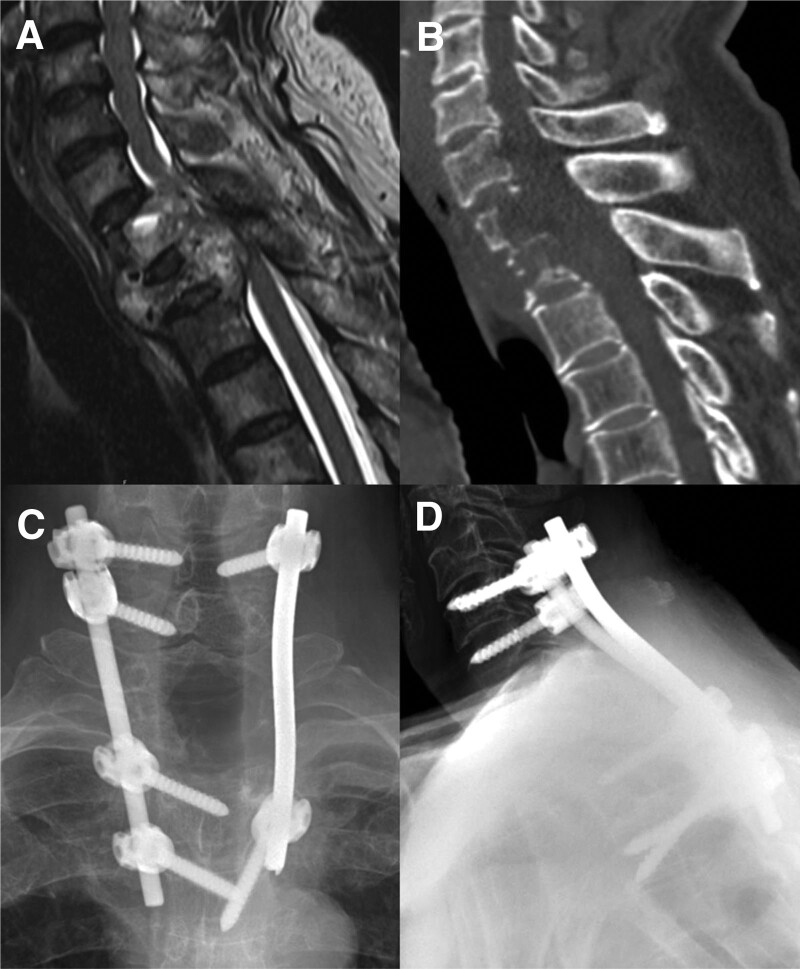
A 64-year-old man diagnosed with kidney cancer presented to the hospital with gait disturbance and severe back pain. Preoperative sagittal MRI (A) and sagittal CT (B) reveals the osteolytic metastatic spinal tumor at T1–2, compromising the spinal cord. Tumor removal with CTJF using 5.5-mm-diameter rods is performed from C6 to T4. Postoperative anteroposterior (C) and lateral (D) X-ray films show well-fixed pedicle screws at the cervicothoracic junction. CT = computed tomography, CTJF = cervicothoracic junction fixation, MRI = magnetic resonance image.
